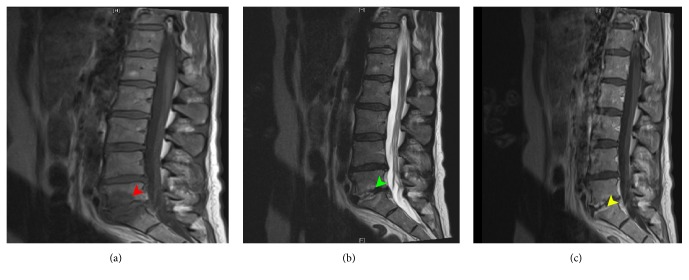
Sagittal MRI pulse sequences of lumbosacral spine at presentation. (a) (T1-weighted) shows markedly reduced signal at the L5-S1 level while (b) (T2-weighted) shows increased T2 signal both in keeping with edema. (c) shows enhancement of the vertebral end plates. All findings are in keeping with discitis.
